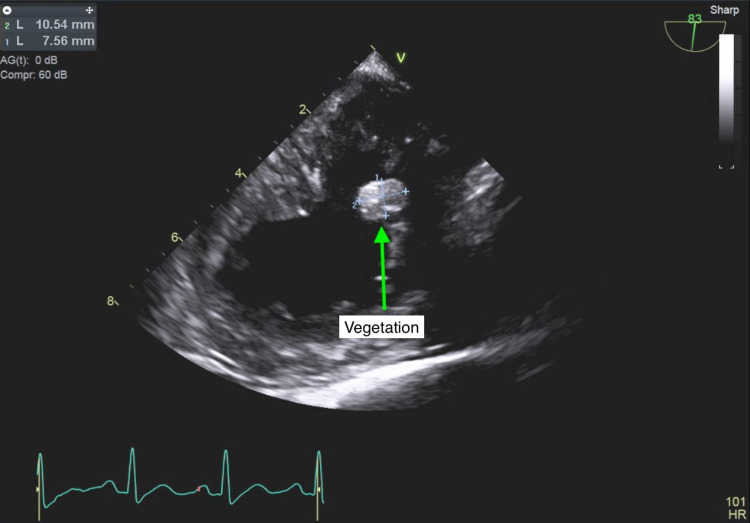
Transesophageal echocardiogram showing a 10 mm echodensity attached to the anterior mitral valve leaflet.
